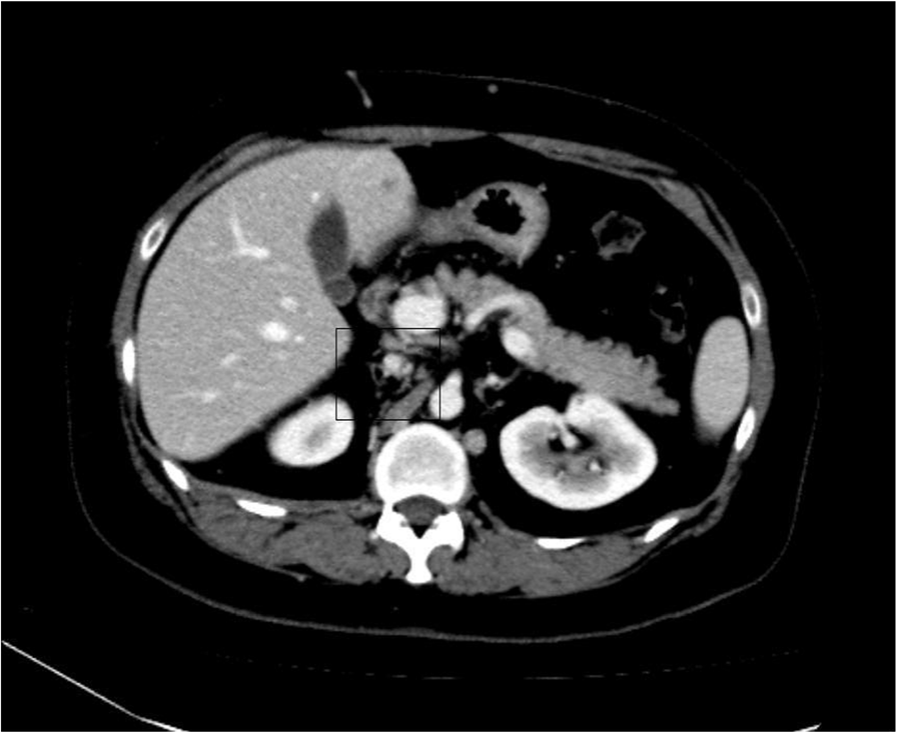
Computed tomography scan showing the narrowed inferior vena cava distal to the liver and dilated collaterals on the anterior abdominal wall (boxed region).
